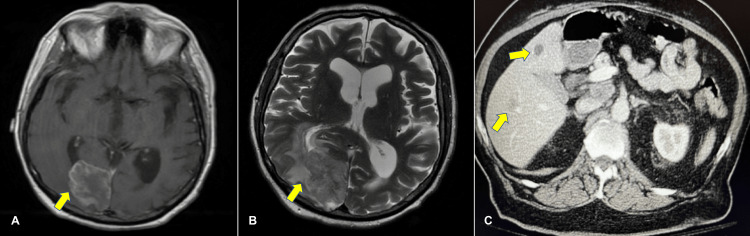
Brain MRI images A. MRI of the brain, axial view. B. MRI of the brain, horizontal view. C. CT scan of the abdomen with two lesions in the liver. CT, computed tomography; MRI, magnetic resonance imaging
